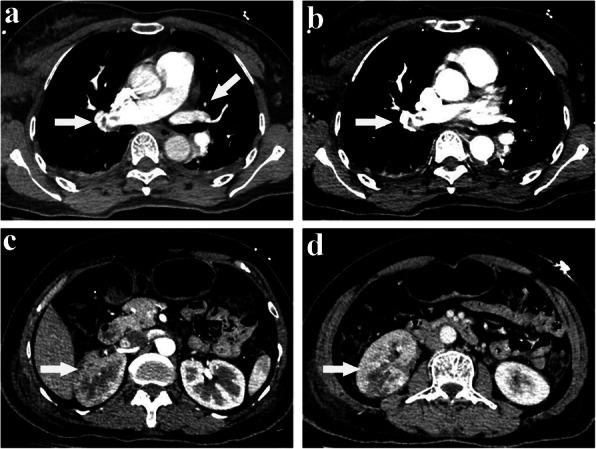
After seven days treatment of low molecular heparin anticoagulant therapy, Contrast-enhanced CT angiography revealed that most previous invisible thrombus in main bilateral branches of pulmonary trunk was dissolved and disappeared (a and b). Simultaneously, the blood flow perfusion of right kidney was restored without renal insufficiency (c and d)
